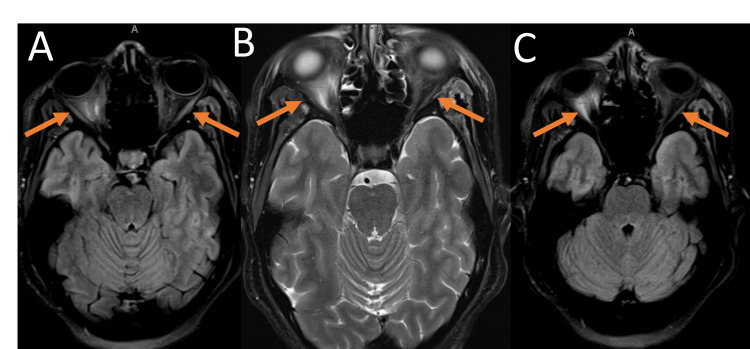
Multiple MRI without contrast highlighting bilateral orbits Figure 2A shows FLAIR MRI without contrast abnormal signal intensity in the intraconal orbits with mild edema and enhancement of the optic nerve sheath complexes bilaterally and enhancement of the posterior uveal scleral margin pointed by orange arrows. Figure 2B demonstrates T2-weighted MRI without contrast idiopathic orbital inflammation or a manifestation of an infectious or noninfectious systemic inflammatory disease shown by orange arrows. Figure 2C shows FLAIR MRI, which also highlights the orbital inflammatory changes in orange arrows.
